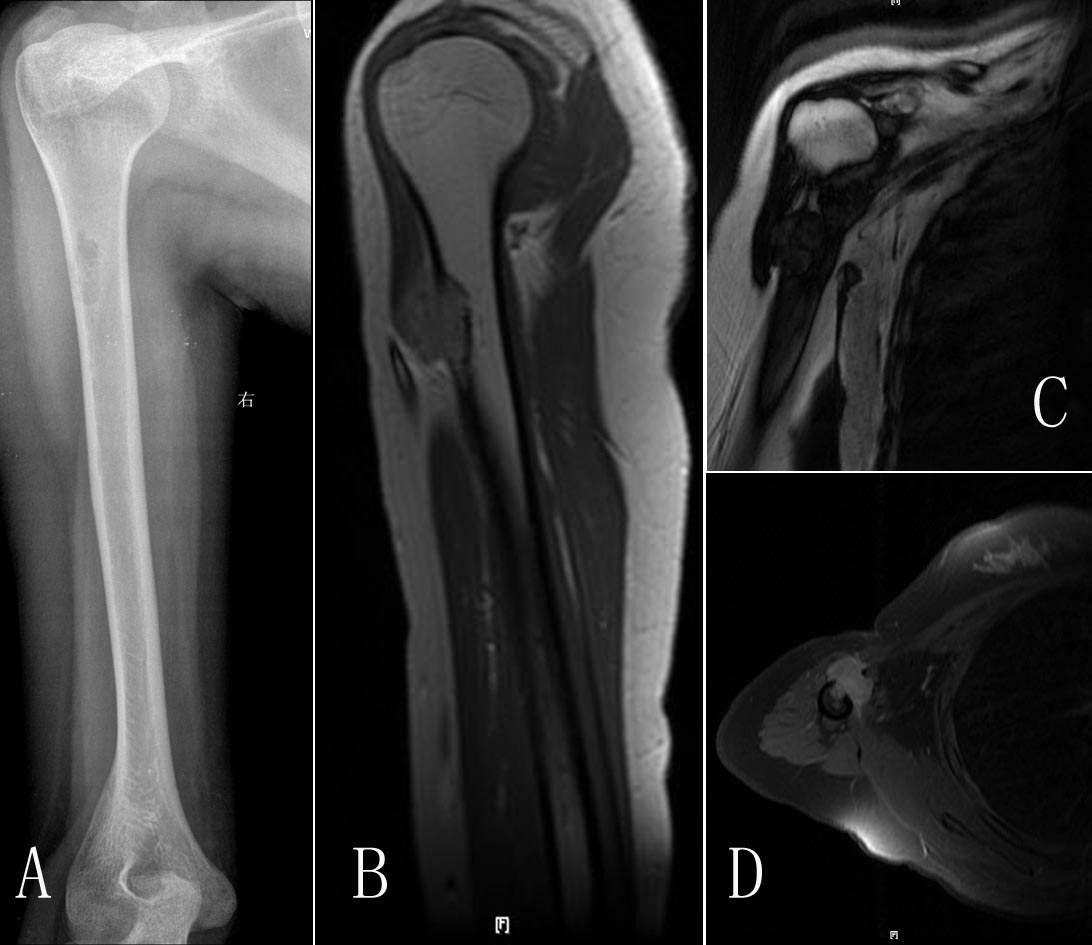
A, Radiographs show an ill-defined, destructive, osteolytic lesion of the right proximal humerus. B, C, D T1, T2 and axial view MR images show an irregularly shaped extraosseous mass extending around the right humerus.
